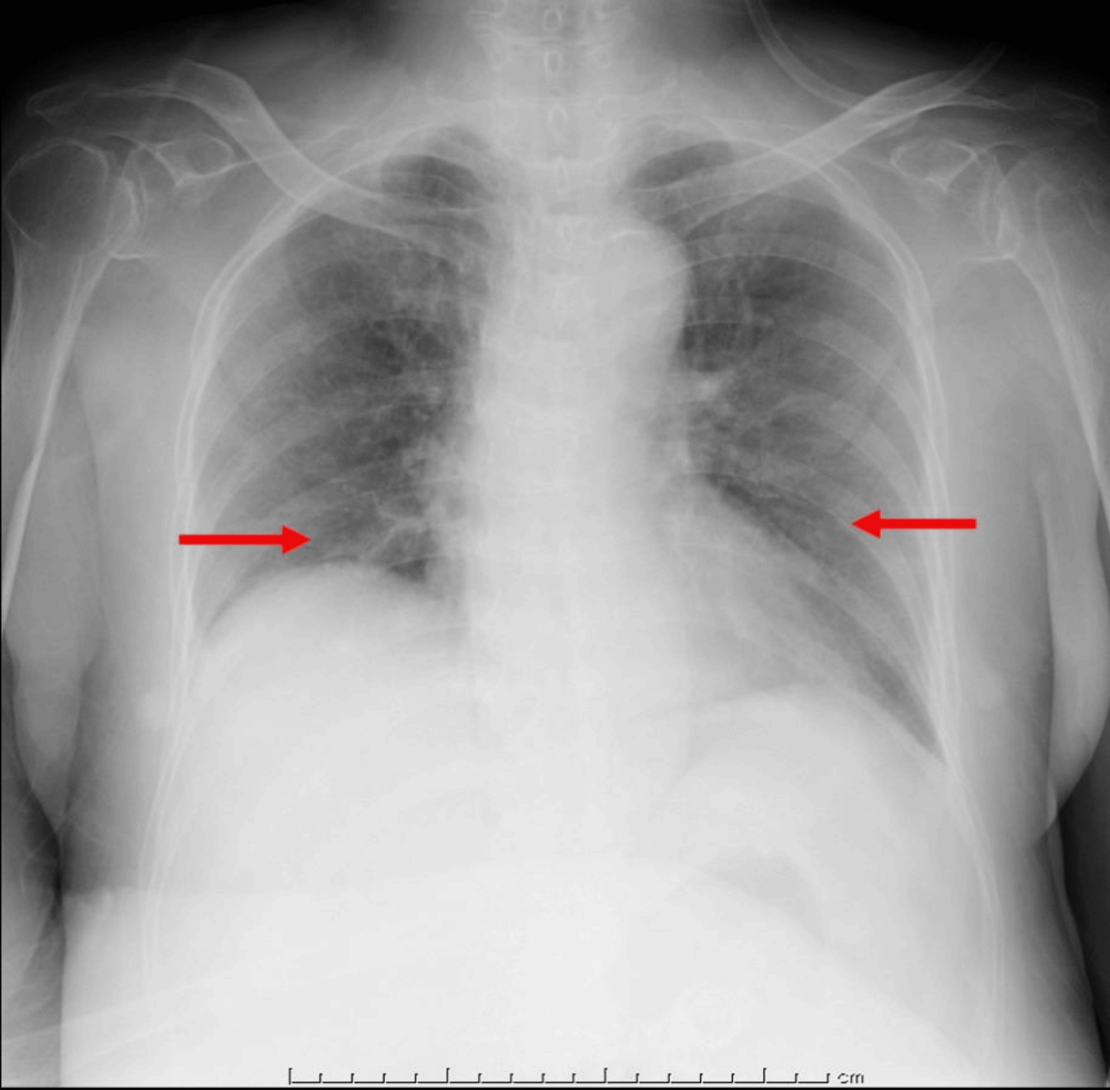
Chest X-ray The anterior-posterior X-ray view of the chest. The arrows indicate the ground glass shadows of both lung fields.
